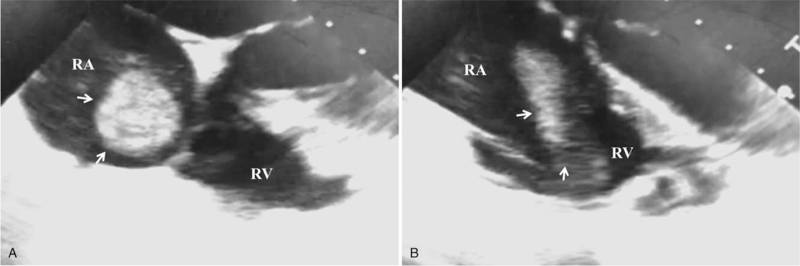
(A) Arrows indicate large right atrial mass via transesophageal echocardiography. (B) Mid-esophageal 4 chamber view shows the mass (arrow) protruding into the right ventricle. RA = right atrium, RV = right ventricle.
